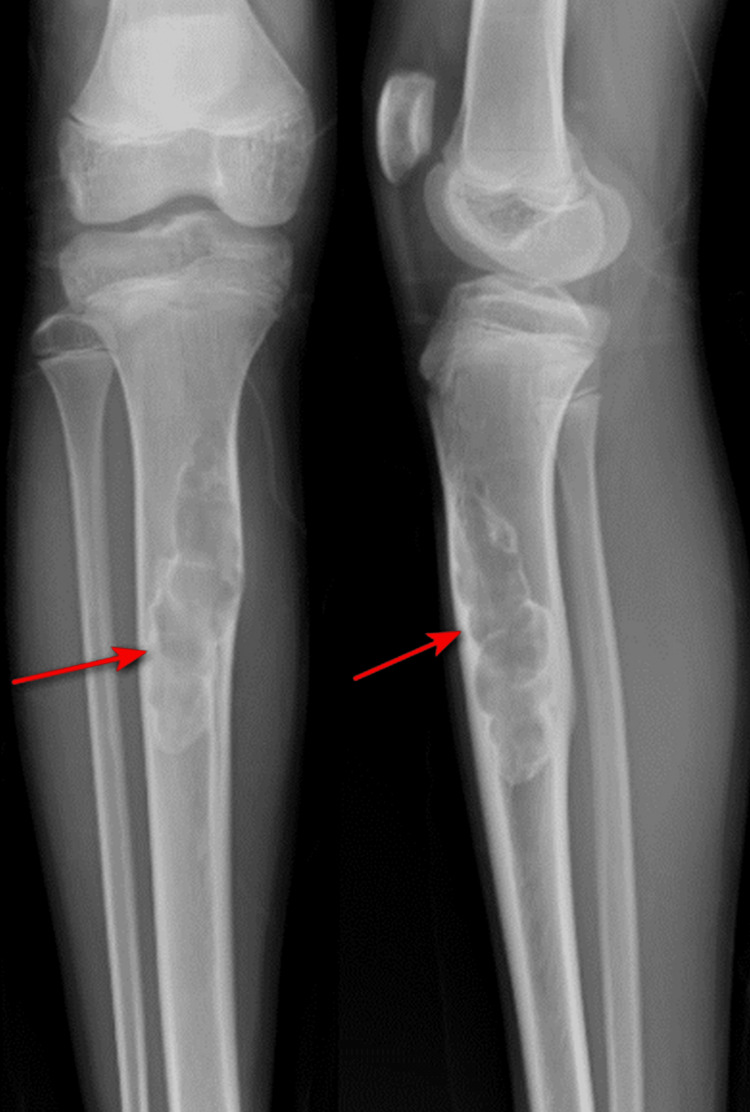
Anteroposterior and lateral plain radiographs of the right lower limb showed a well-defined, expansile lytic-sclerotic lesion (red arrow).
